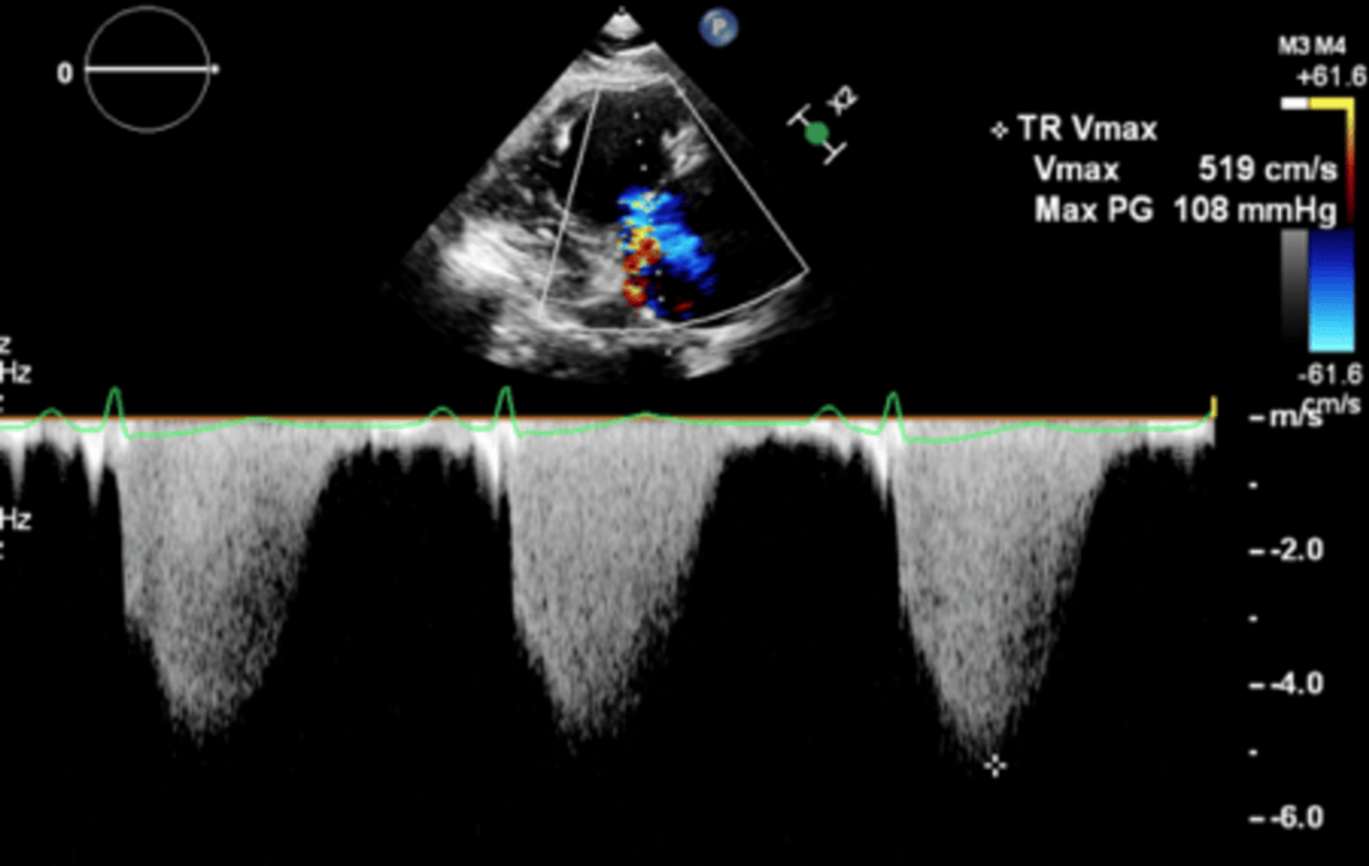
Transthoracic echocardiography showing the maximum velocity of the tricuspid regurgitation jet as 5.19 m/s and the maximum gradient as 108 mmHg. The RA pressure is 8 mmHg. The calculated PA systolic pressure is 116 mmHg (PASP = 4 X TRV2 + RA pressure). PA, pulmonary artery; PASP, pulmonary artery systolic pressure; RA, right atrium;TRV, tricuspid regurgitation velocity
